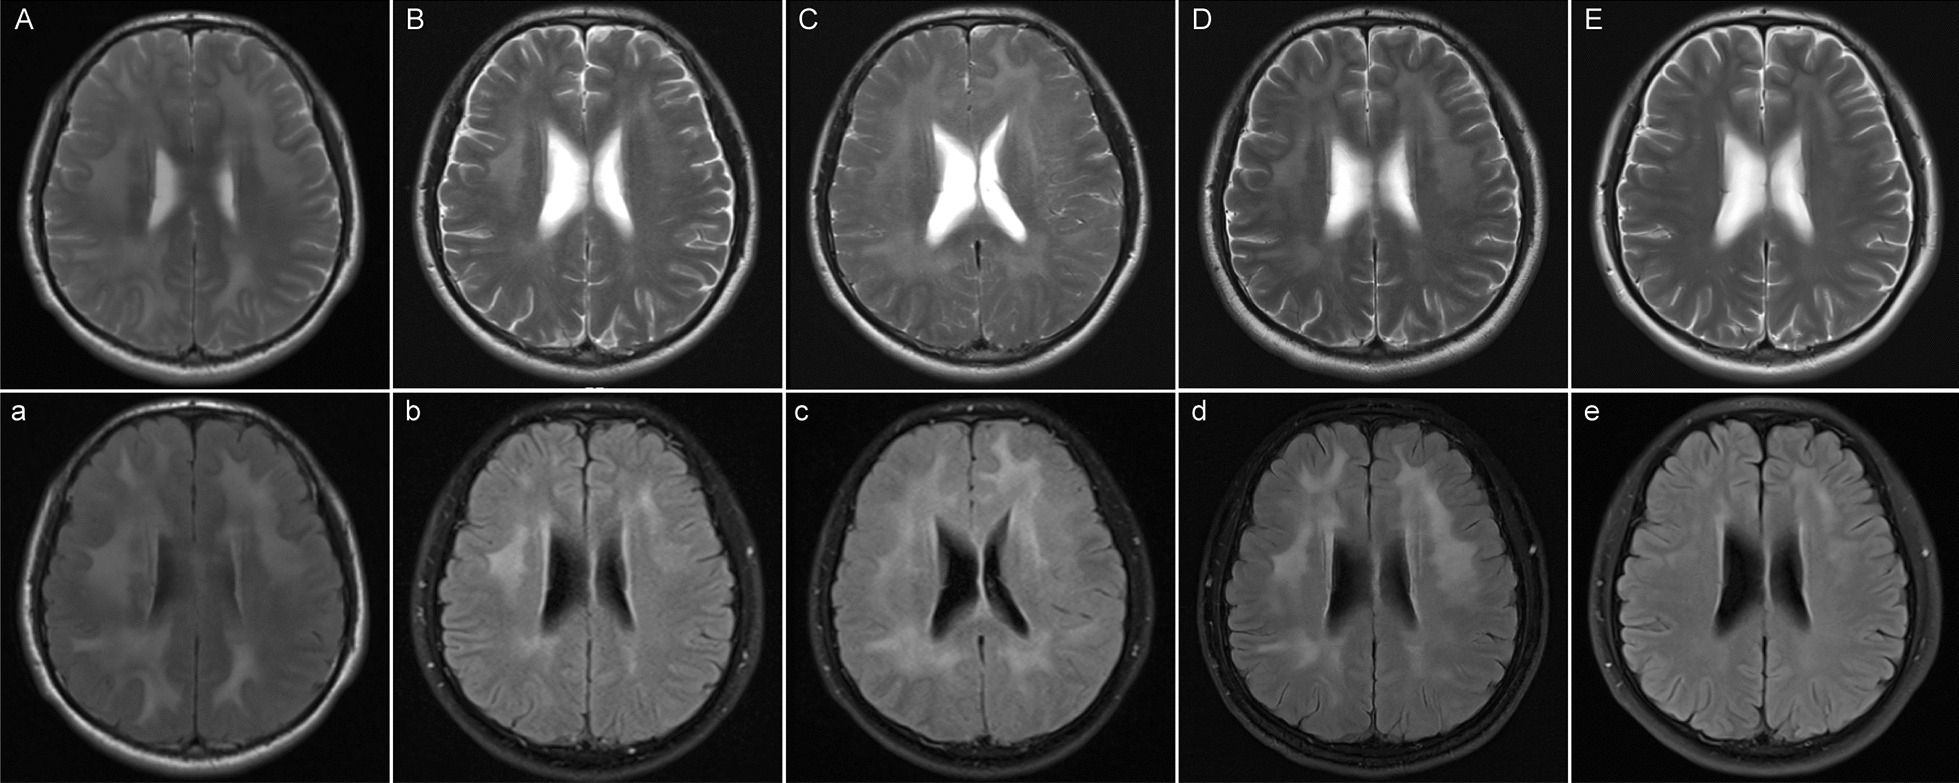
Cerebral MRI, laboratory examinations and treatment during each visit. T2-weighted (A) and T2-FLAIR images (a) on January 8th, 2022 showed diffused high signals of white matter under the bilateral frontoparietal temporal cortex, around the anterior and posterior corners of the lateral ventricles. T2-weighted (B) and T2-FLAIR images (b) on February 17th, 2022 presented obviously reduced area of multifocal leukoencephalopathy. T2-weighted (C) and T2-FLAIR images (c) on June 27th, 2022 showed significantly enlarged diffused white matter high signals on T2 and FLAIR sequence. T2-weighted (D) and T2-FLAIR images (d) on August 20th, 2022 showed slightly reduced affected brain area. T2-weighted (E) and T2-FLAIR images (e) on December 28th, 2022 presented remarkable improvement, leaving only slight white matter damage
